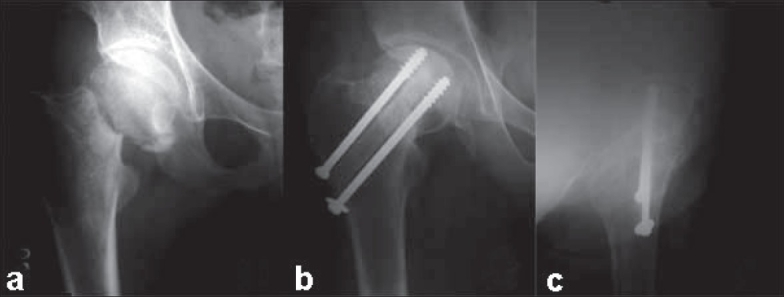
(a) Anteroposterior view of the right hip of a neglected femoral neck fracture (17 weeks old). Follow-up radiograph (anteroposterior (b) and lateral view (c)) showing union with partial incorporation of graft at the end of 30 months
